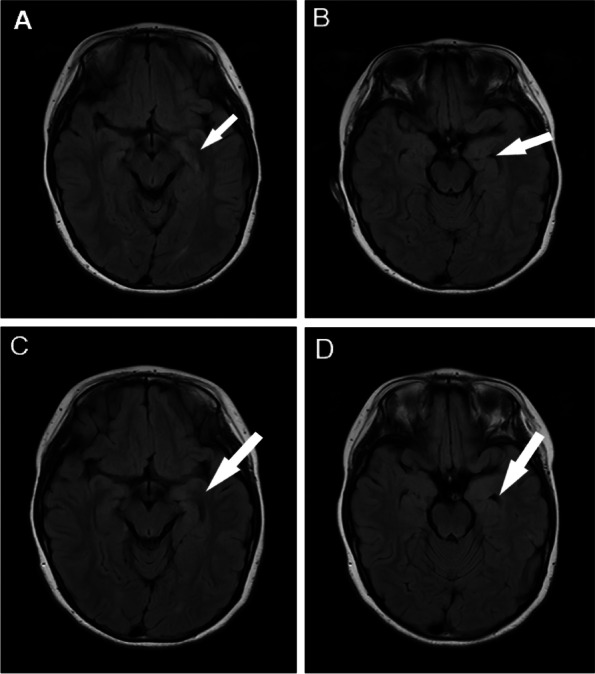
The results of brain MRI. A, B Brain MRI showed increased signal in the left temporal pole before treatment. C, D Brain MRI showed increased signal in the left temporal pole after intravenous immunoglobulin and corticosteroids therapy. Picture A was compared with picture C, the high signal slightly decreased and the edema slightly subsided in the left temporal pole (the white arrow) on T2FLAIR. Picture B was compared with picture D, the temporal horn (the white arrow) was enlarged in the picture D. It indicated that edema subsided after treatment
